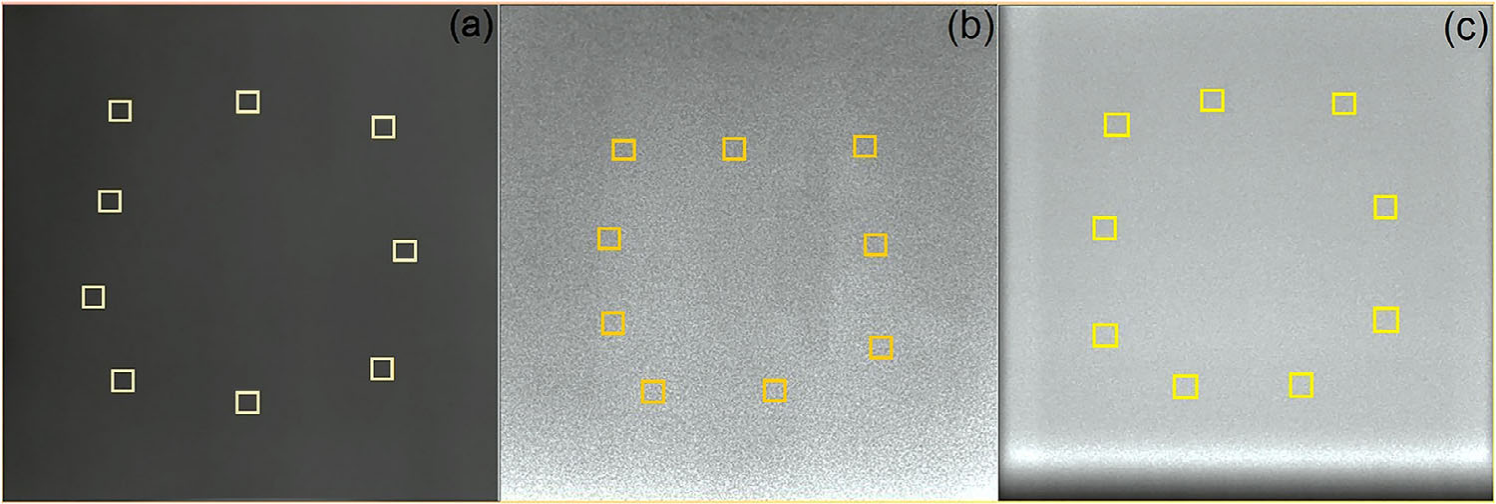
Images for uniformity assessment. Representative uniformity images of (a) CBCT, (b) DR1, and (c) DR2. DR1 and DR2 are the flat panel detectors of the dual‐panel DR system.
